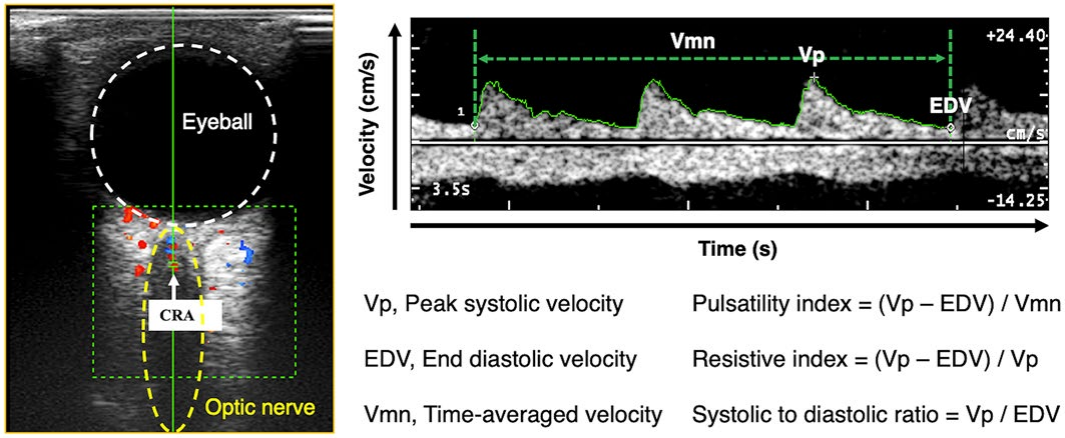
Measurement location and typical waveform of the central retinal artery (CRA) in color Doppler ultrasonography. The locations of the eyeball and optic nerve are outlined by the white circle and yellow ellipse, respectively. Blood flow signals (blue and red areas) appear within the green rectangle. Hemodynamic parameters, including peak systolic velocity (Vp), end diastolic velocity (EDV), time-averaged mean velocity (Vmn), pulsatility index (PI), resistive index (RI) and systolic / diastolic ratio (S/D), were calculated for each triple-waveform.
